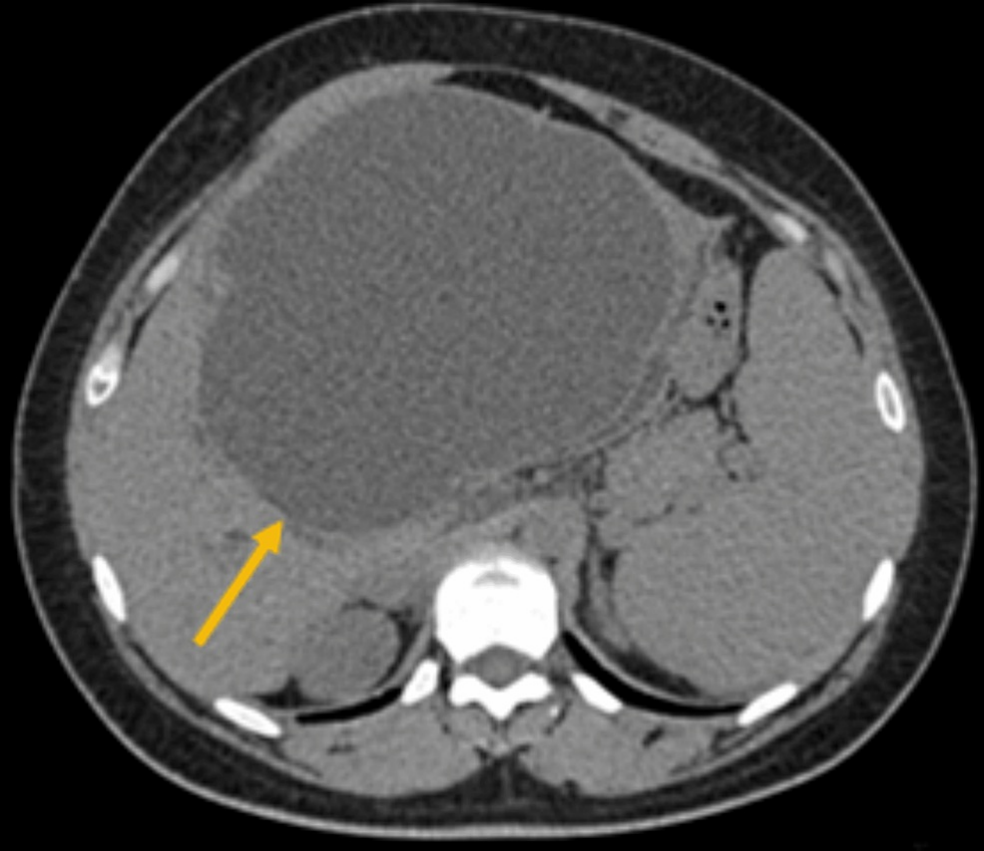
Axial view of computed tomography (CT) of the abdomen The CT scan shows a large hepatic cyst measured 13*17*20 cm seen arising from the left hepatic lobe with a double-wall sign and suspicion of focus of calcification.
